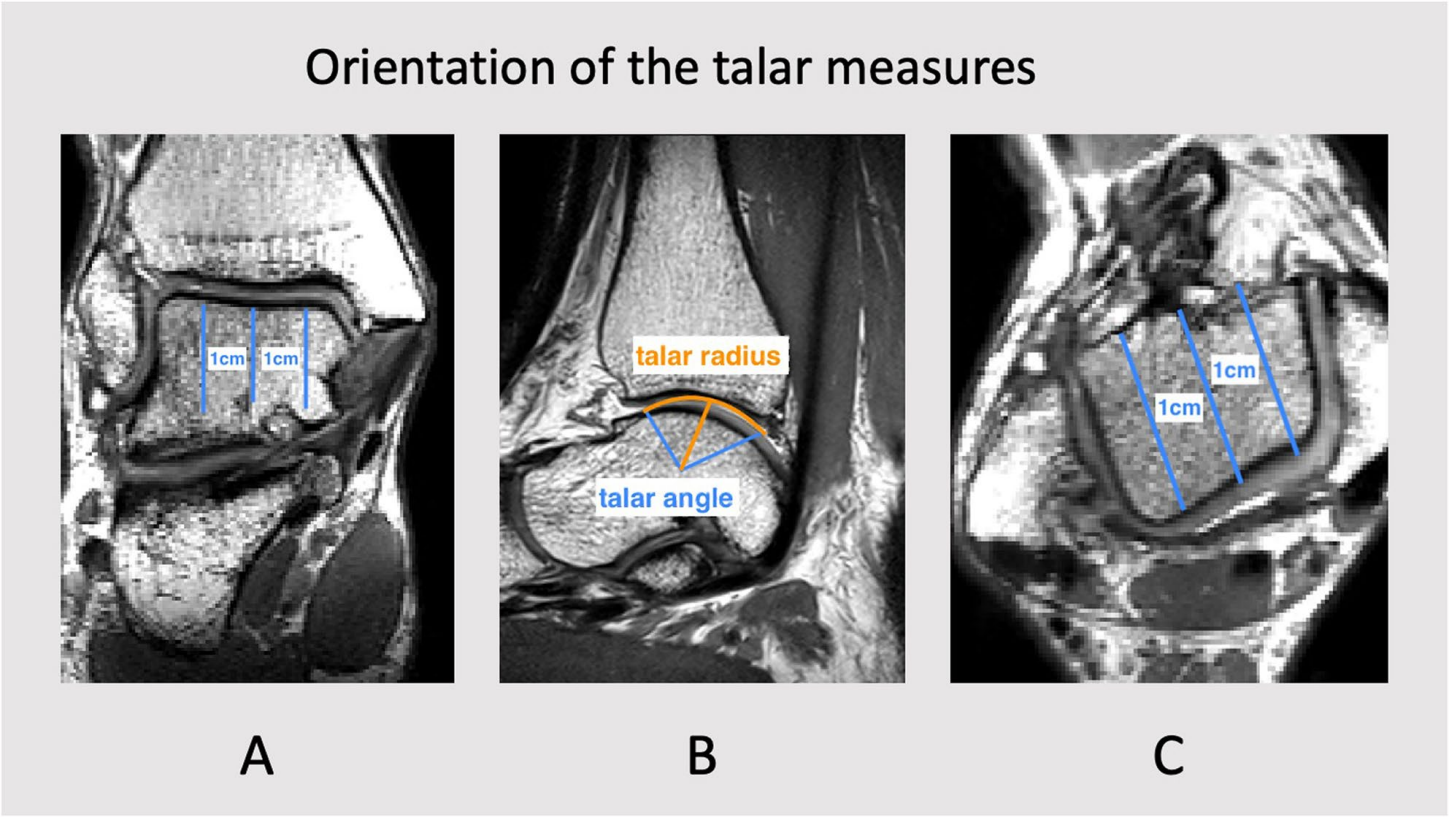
Display of the orientation of the talar measures in an excerpt of a 3D SPACE MRI. A coronar view, B sagittal view, C transversal view
